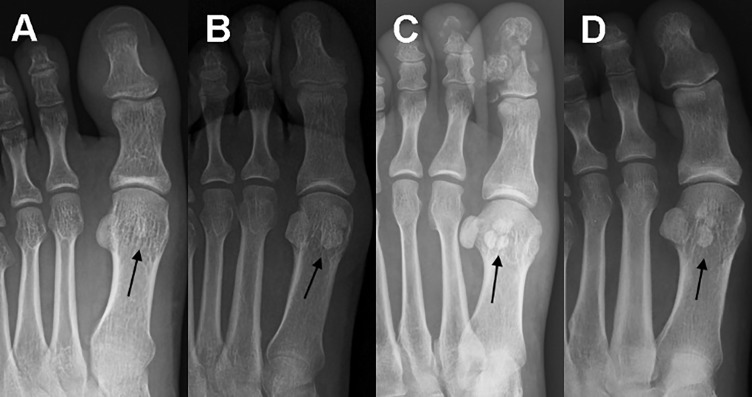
Anteroposterior radiographs of the forefoot of three adults, showing morphological variation among sesamoid bones, identified with the arrow (black): (A) Type I, one ossification center and hypoplastic sesamoid; (B) Type II, two ossification centers; and (C and D) Type III, ≥ three ossification centers.
